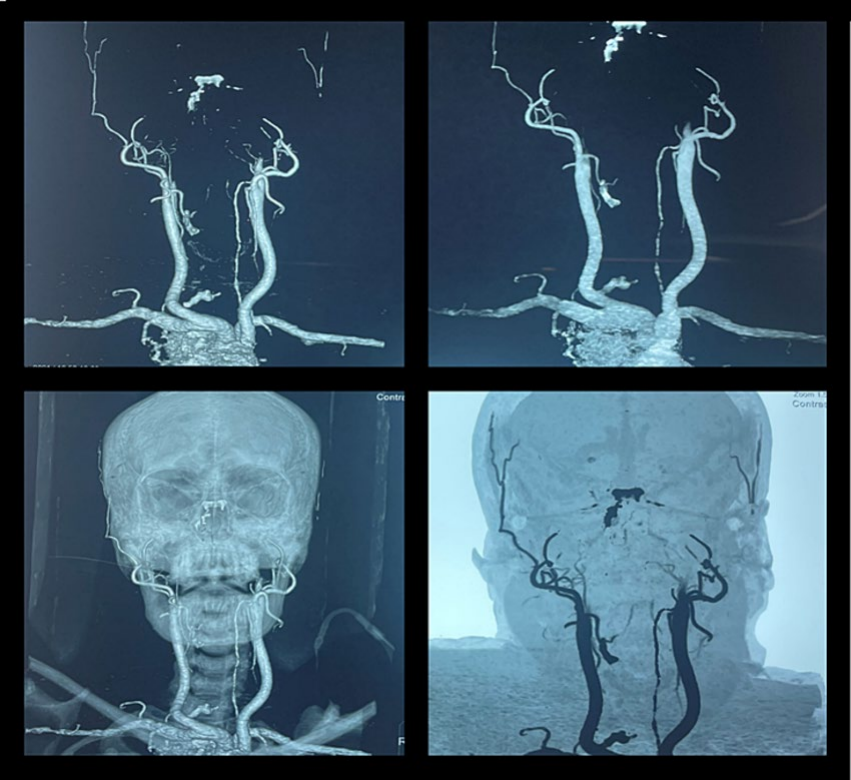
CT angiography in brain death. A 46-year-old woman was brought to our emergency department after a fatal motor vehicle accident. Her Glasgow Coma Scale (GCS) score was three on arrival. She had bradycardia and hypotension. The CT scan of the head showed a massive subdural hematoma with mass effect and compression of the brainstem with signs of herniation. She was intubated to secure the airway, and ICP was monitored by a subdural electrode. The raised ICP remained refractory to standard medical therapy, and her neurologic status progressively deteriorated and remained unresponsive. She further developed fixed dilated pupils, and her clinical findings were suggestive of brain death. The CT angiography showed non-opacification of the intracranial arteries beyond the internal carotid artery, suggestive of brain death.
